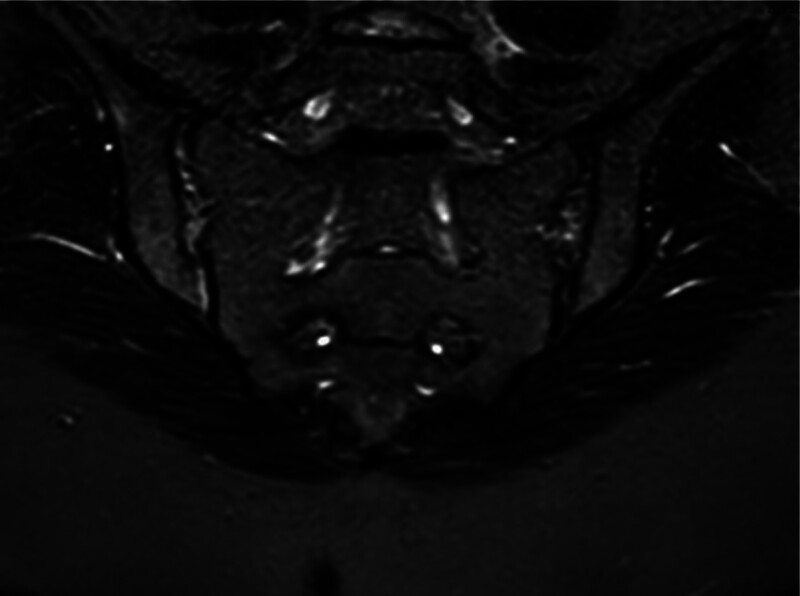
Axial fat-suppressed T2-weighted MRI of the sacroiliac joints showing subchondral bone marrow edema, compatible with active sacroiliitis. MRI = magnetic resonance imaging.
